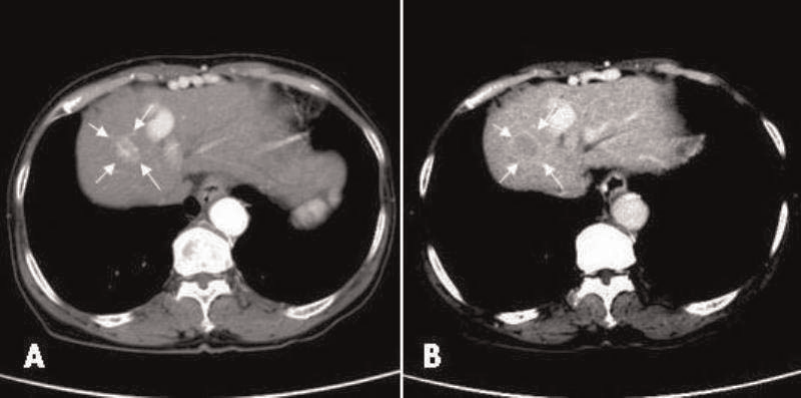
Axial computed tomography image with contrast enhancement before angiography. Classical HCC (20 mm) was observed in segment 4 (arrows). Collateral veins were seen along the abdominal wall (arrow heads). A; Early phase. B; Delayed phase.
